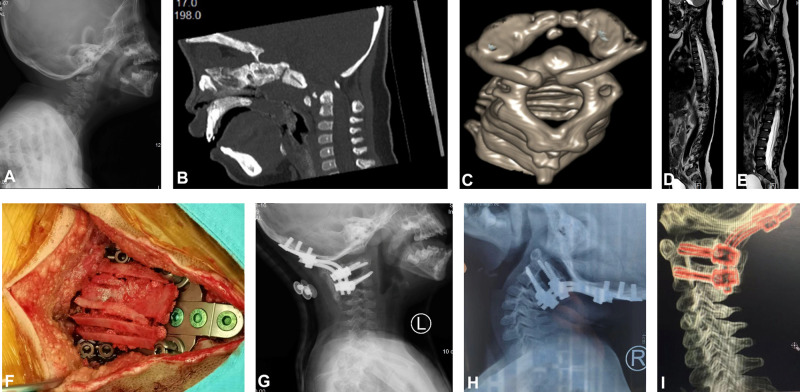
Case 2. A 3-year-old female child with DS, atlantoaxial dislocation, OsO, and spinal cord injury. (A) Dynamic X-ray showed that the ADI increased significantly, and a shift of the atlas anterior and lower to the axis. (B) Cervical sagittal reconstruction computed tomography (CT) and (C) three-dimensional reconstruction showed atlantoaxial dislocation with OsO. (D and E) Magnetic resonance imaging (MRI) showed that the upper cervical spinal cord was compressed by the upper posterior part of the odontoid process, and the spinal cord was edematous and degenerated. (F) Reduction was performed under cranial traction during surgery. (G) Postoperative X-ray films showed atlantoaxial screw rod fixation. (H and I) At postoperative 1 year, atlantoaxial CT and X-ray showed good atlantoaxial fusion and satisfactory internal fixation position.
